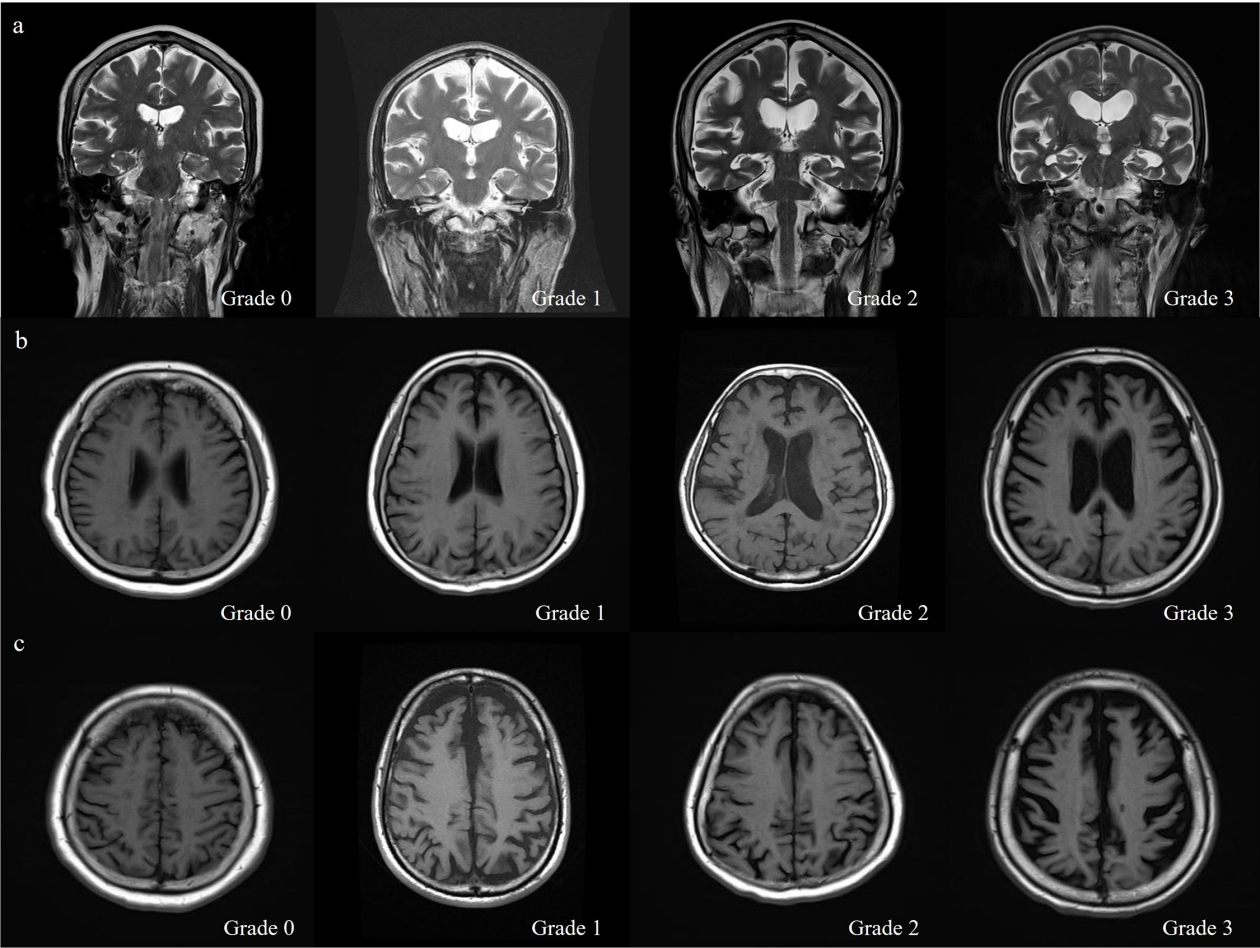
Brain atrophy in structural MRI using visual rating scales. (A) Medial temporal atrophy according to Scheltens scale with oblique coronal T2-weighted images. (B) Global cortical atrophy graded on axial FLAIR images. (C) Parietal atrophy scored using the posterior cortical atrophy scale with axial FLAIR images. *FLAIR*: *fluid-attenuated inversion recovery*.
